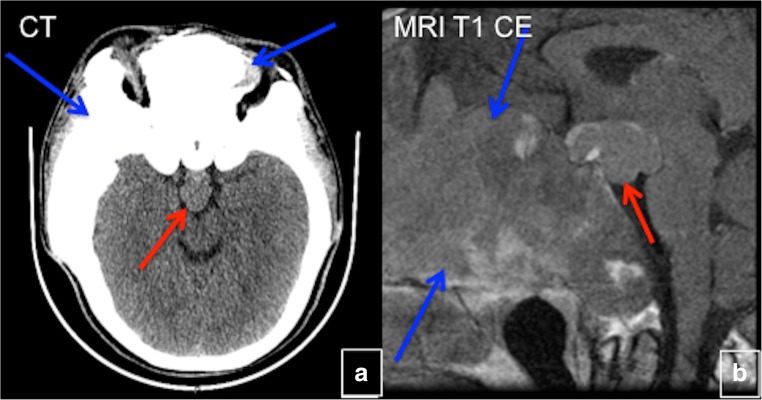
Pituitary adenoma in craniofacial fibrous dysplasia (FD). a, b A patient with craniofacial FD (*blue arrows*) with pituitary macroadenoma (*red arrows*) on CT (a) and T1 contrast-enhanced MRI (b)
